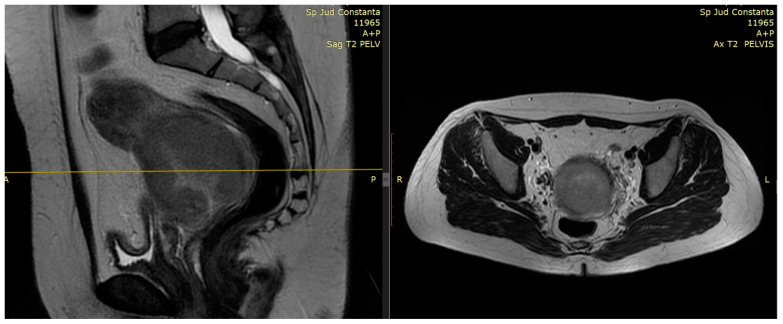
Patient 1 with FIGO stage IIIC2 was treated with EBRT and 3 fractions of HDR CT-based brachytherapy. T2-weighted pretreatment MRI with 6.8 × 6 × 6.2 cm hypointense vaginal wall tumor and superior 1/3 vaginal invasion, bilateral parametrial involvement—sagittal view and axial view.
